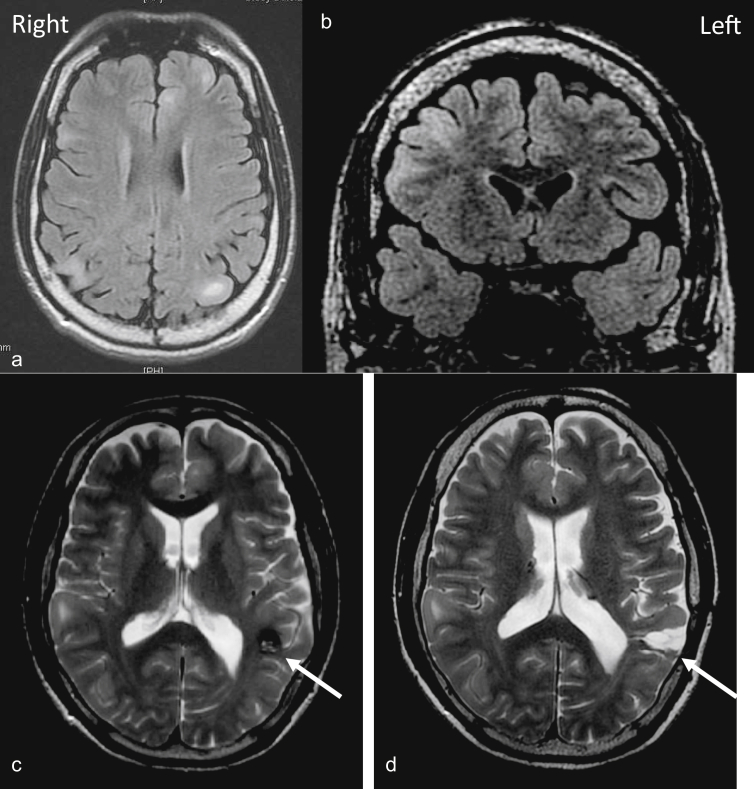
Multiple cortical tubers and pre-/post-surgical magnetic resonance image (MRI). The fluid-attenuated inversion recovery (FLAIR) MRI shows multiple tubers (a and b). The T2-weighted MRI shows the pre- (c) and post- (d) removal of the CA (arrow).
